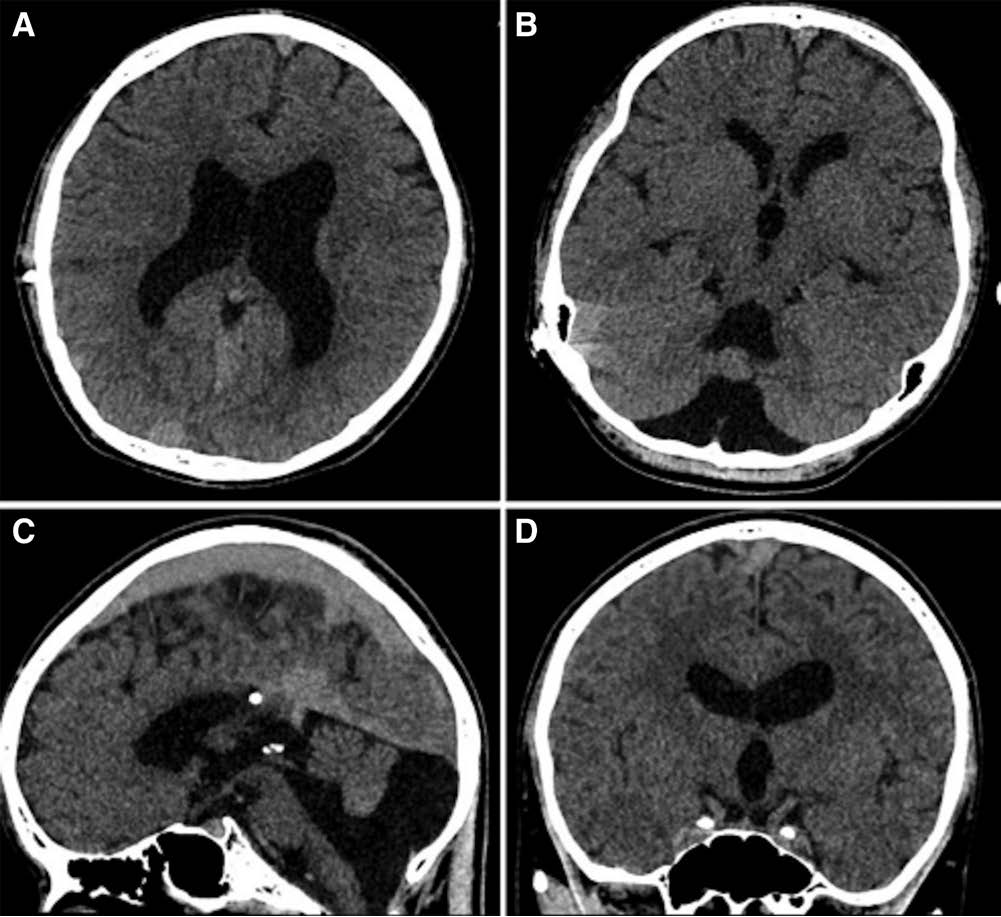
MR images of the patient (July 8, 2021). (a) An enlarged bilateral ventricle. (b/c) Hypoplasia of the cerebellar vermis, cystic dilatation of the fourth ventricle, enlarged posterior fossa with upward displacement of sinus confluens. (d) Dilatation of the cerebral ventricle and the third ventricle. MRI = magnetic resonance imaging.
